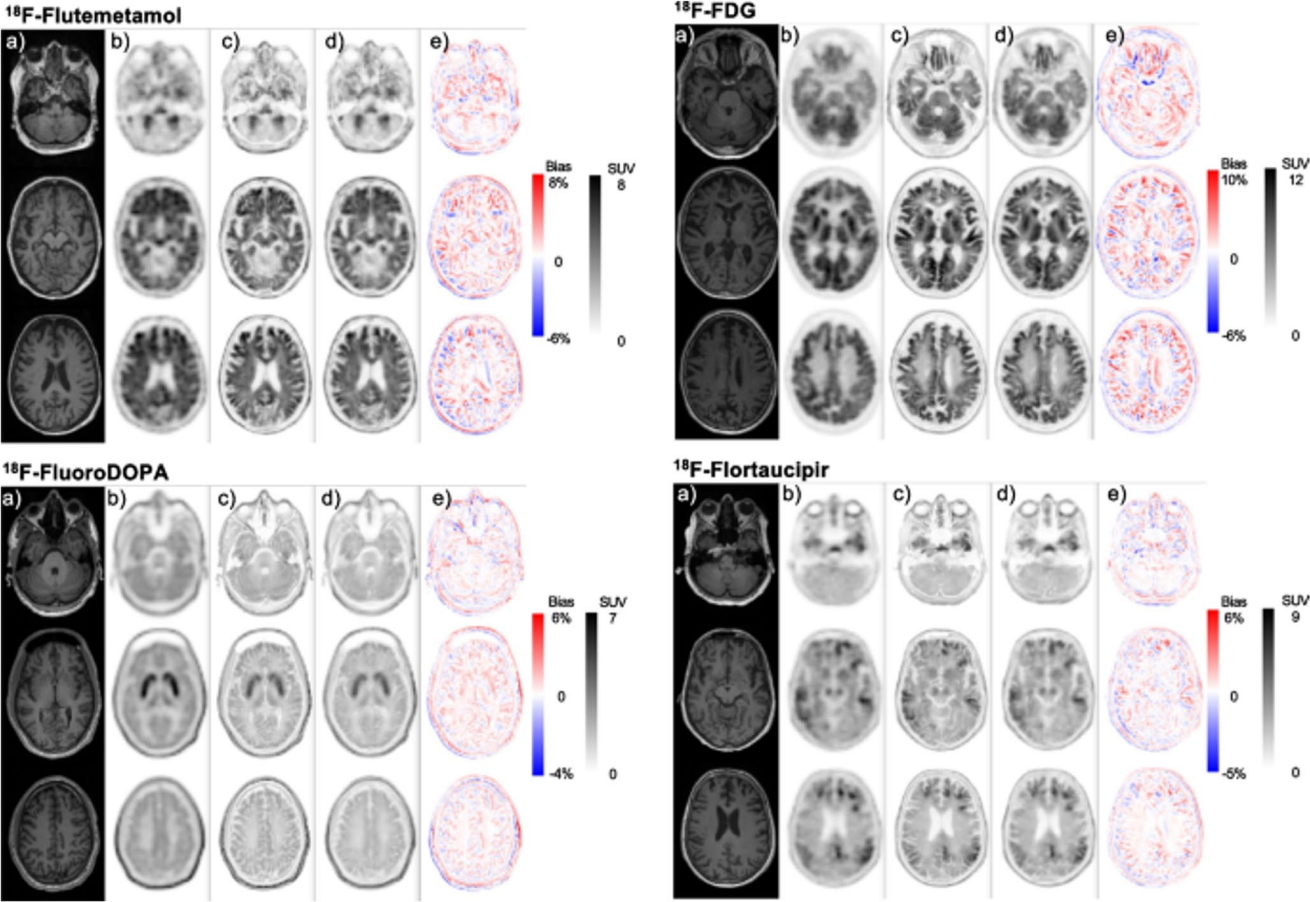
Example slices from multi-tracer brain PET scans of various patients showcasing: (a) co-registered T1-weighted MRI, (b) non-PVC PET images, (c) MRI-guided reference PVC PET images, (d) DL-predicted PVC PET images, and (e) corresponding bias maps. These results highlight the model’s ability to reproduce reference PVC image quality while maintaining tracer-specific uptake patterns. Reprinted with permission from [214] under a Creative Commons Attribution License (CC BY 4.0 DEED)
